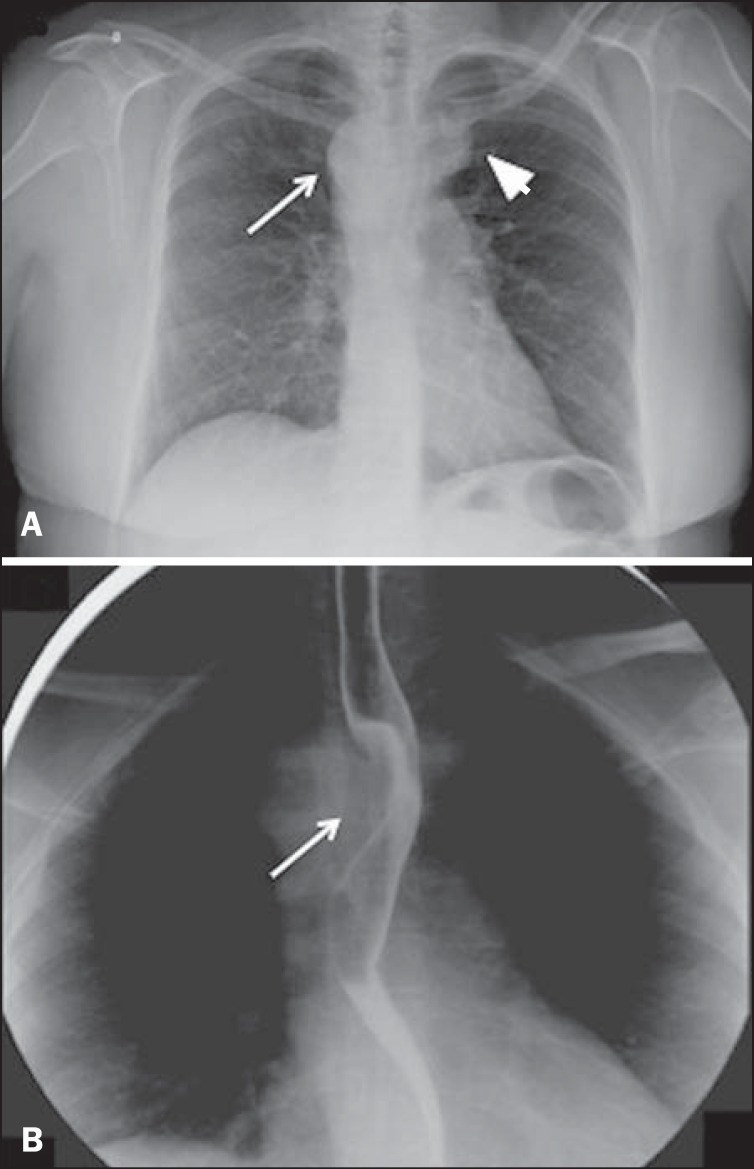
A: Posteroanterior chest X-ray showing right aortic arch
